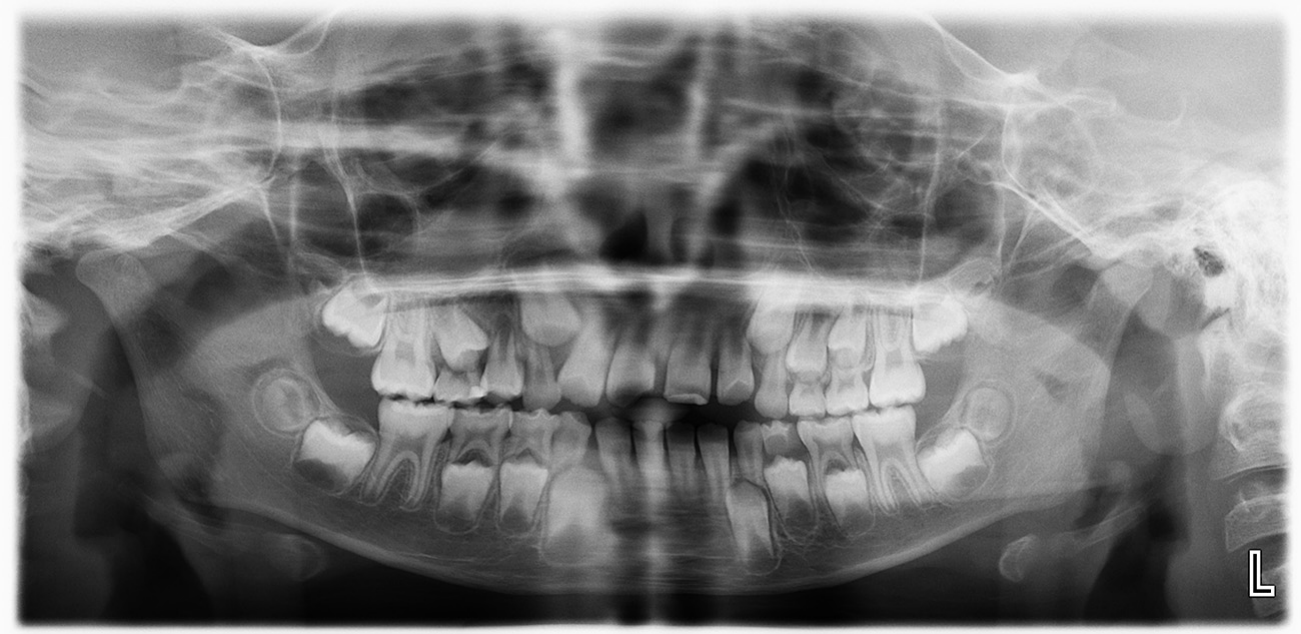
Preoperative panoramic view radiograph.
